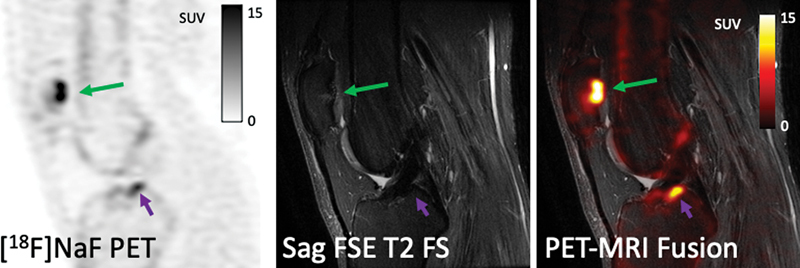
A 29-year-old man (body mass index: 26.3) with posttraumatic osteoarthritis (9 years after anterior cruciate ligament tear and reconstructive surgery). Heavily elevated NaF uptake is seen in the patella, correlating with cartilage loss and bone marrow lesion on magnetic resonance imaging (MRI) (green arrow). There is also a highly elevated uptake in the central tibia without any morphological correlate on MRI.
